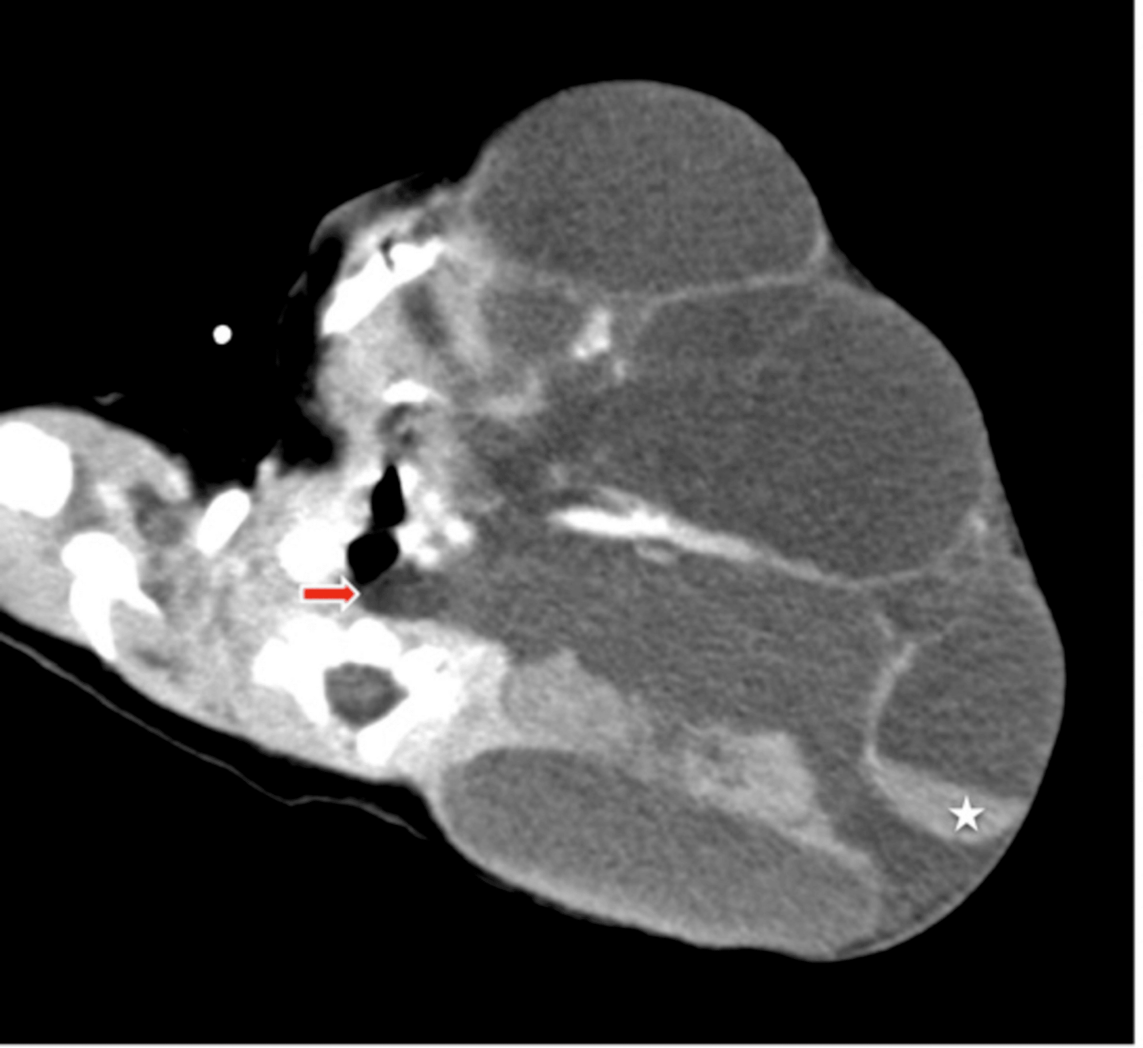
Contrast-enhanced CT image demonstrating a large multiloculated cystic mass in the left cervicofacial region, containing fluid and hemorrhagic components (asterisk). Several septa appear thickened, with internal extension toward the prevertebral space (arrow) and close contact with ipsilateral cervicofacial structures.
